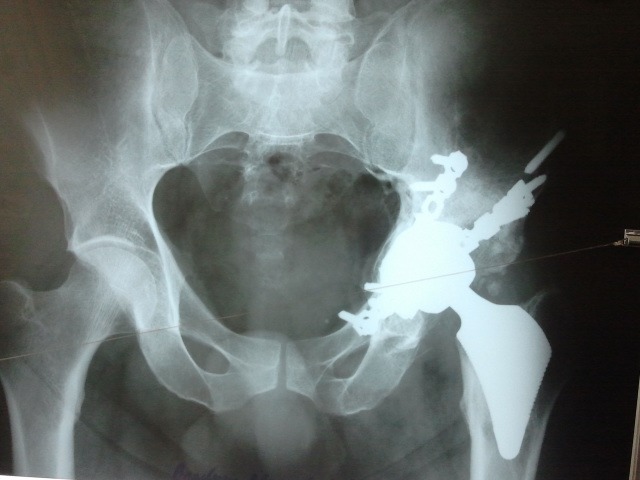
Postoperatory radiographic control
